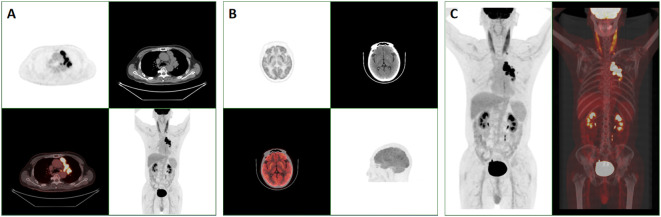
PET-CT was subsequently performed for further evaluation of a lung mass detected on CT. (A) A hypermetabolic mass (SUVmax 9.8) is seen in the anterior segment of the left upper lobe near the hilum. (B) No significant morphological or metabolic abnormalities are identified in the brain. (C) Whole-body imaging shows no evidence of distant metastasis.
